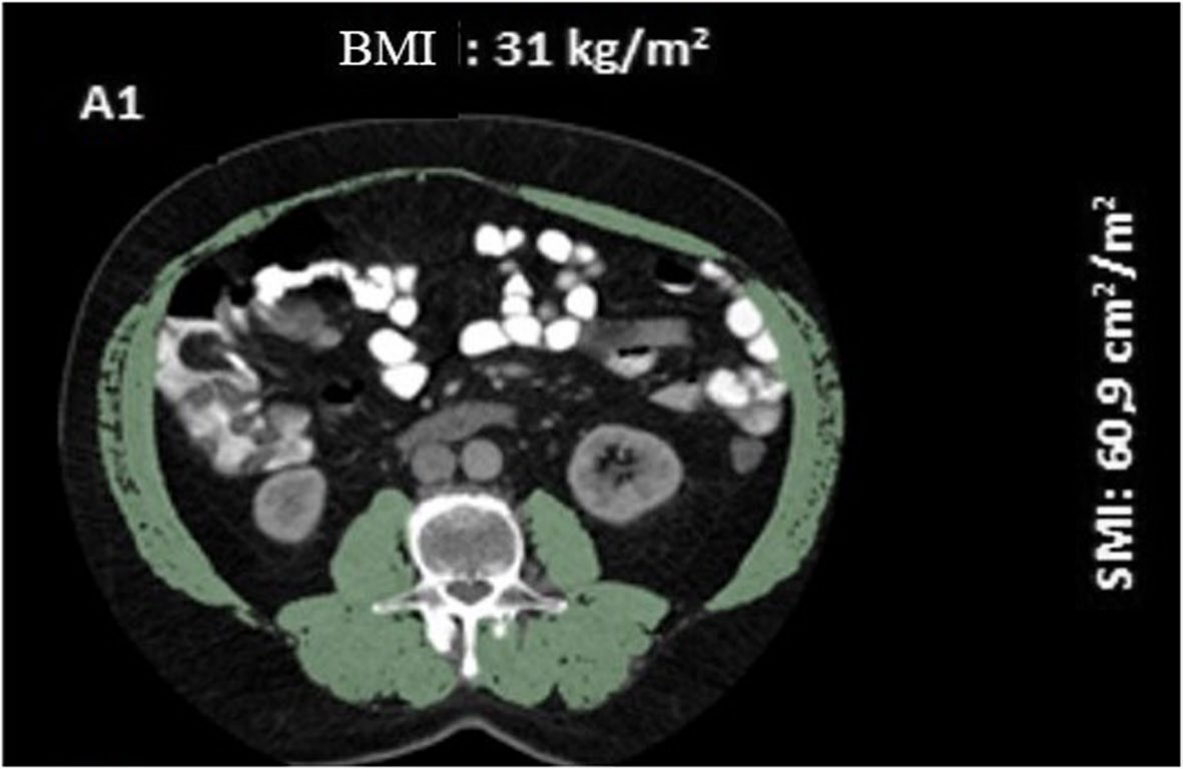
Example of skeletal muscle index measurements on CT sections through the L3 vertebra level. A1: BMI 31 kg/m2 and SMI 60,9 cm2 /m2
